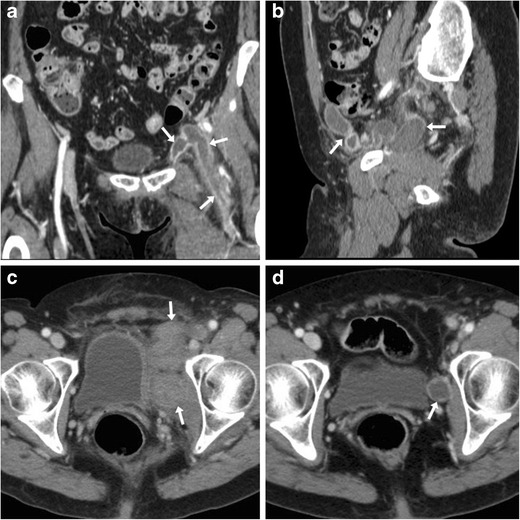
Endovenous heat-induced thrombosis (EHIT) in a 55-year-old woman experiencing left-sided groin pain, tenderness and swelling three days after endovenous laser ablation of the ipsilateral small saphenous vein. Multiplanar contrast-enhanced CT images (A, B) panoramically showed the full extent of iliac-femoral EHIT including the ipsilateral inferior hypogastric and obturator veins, with mild dilatation, luminal non-opacification and prominent enhancement of venous walls (arrows). One week later, repeated CT (C) showed persistent thrombosis of proximal femoral and obturator veins with disappeared mural enhancement (arrows) and increased mass effect on the urinary bladder. Follow-up CT three months later (D) showed recanalisation of the left femoral vein and persistence of chronic obturator vein thrombosis [Adapted from Open Access ref. no. [38]]
